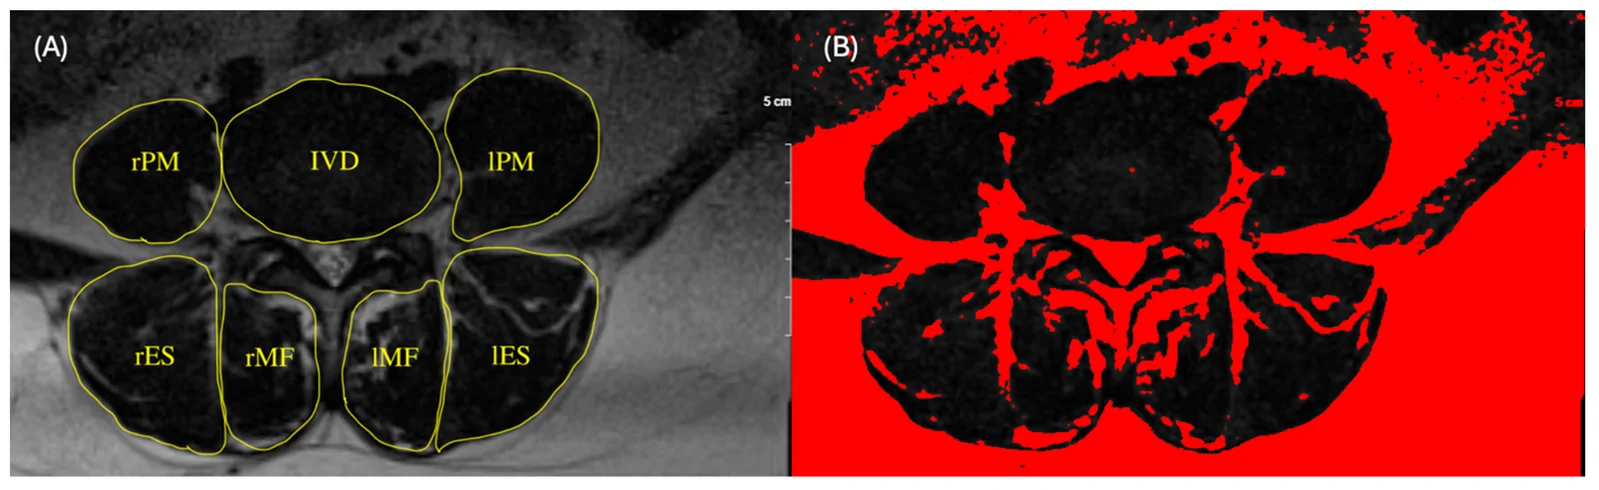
Representative axial T2-weighted lumbar magnetic resonance image demonstrating paraspinal muscle segmentation and fatty infiltration analysis. (A) Manual delineation of the bilateral psoas major (PM), erector spinae (ES), multifidus (MF), and intervertebral disc (IVD) regions of interest. (B) Threshold-based image segmentation used to identify fatty infiltration within the selected regions. Red pixels represent adipose tissue, whereas black pixels represent lean muscle tissue and other non-fat structures. r, right; l, left; PM, psoas major; ES, erector spinae; MF, multifidus; IVD, intervertebral disc.
